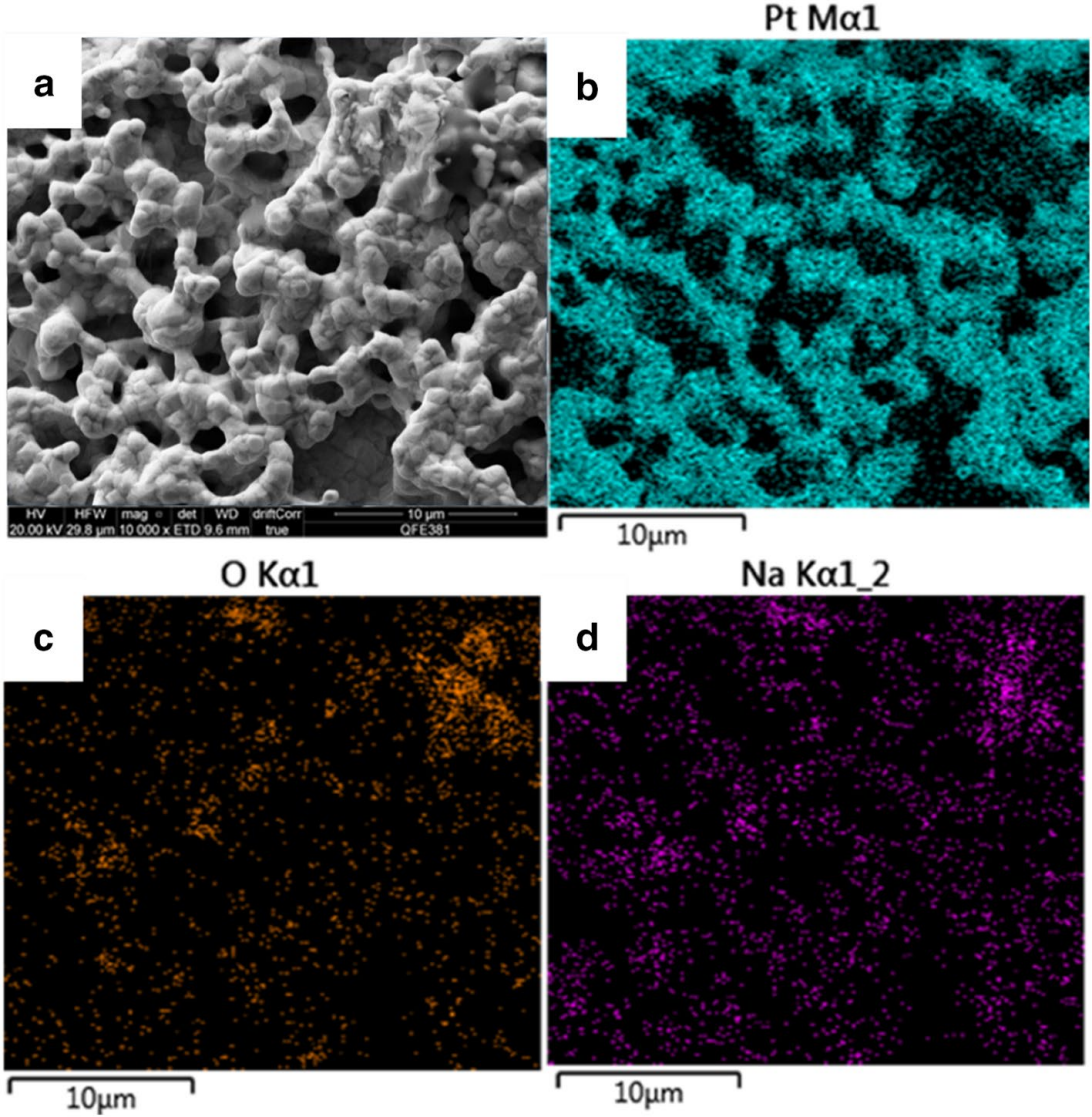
SEM image of the used Na-modified Pt surface with magnification of ×10,000 (a) and the corresponding X-ray mapping of Pt (b), O (c) and Na (d)
